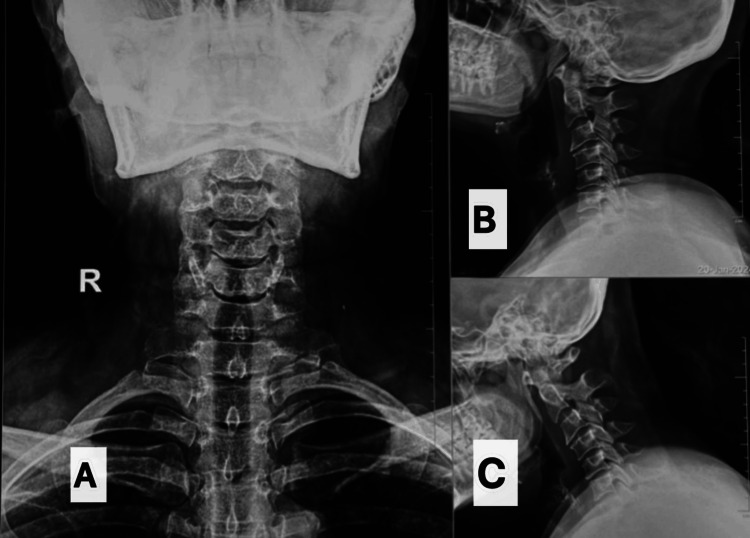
Preoperative cervical anteroposterior (A) and lateral-extension (B)/flexion (C) radiographs showing marginal osteophytes at C3-4 and C4-5 levels with normal disc height and no instability. C3-4: cervical 3-4, C4-5: cervical 4-5
